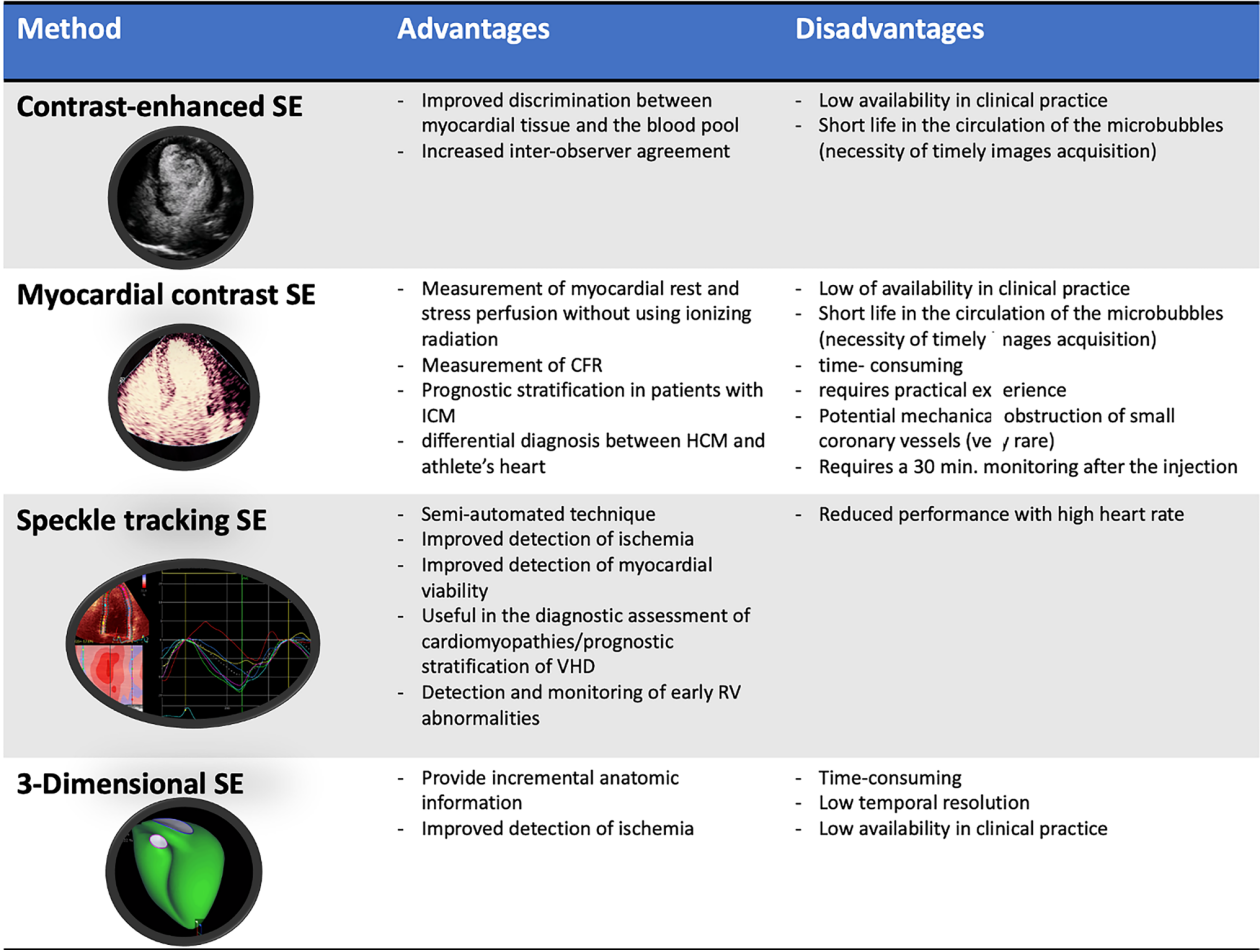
New perspectives in stress echocardiography. CFR, coronary flow reserve; HCM, hypertrophic cardiomyopathy; ICM, ischemic cardiomyopathy; RV, right ventricle; SE, stress echocardiography; VHD, valvular heart disease
